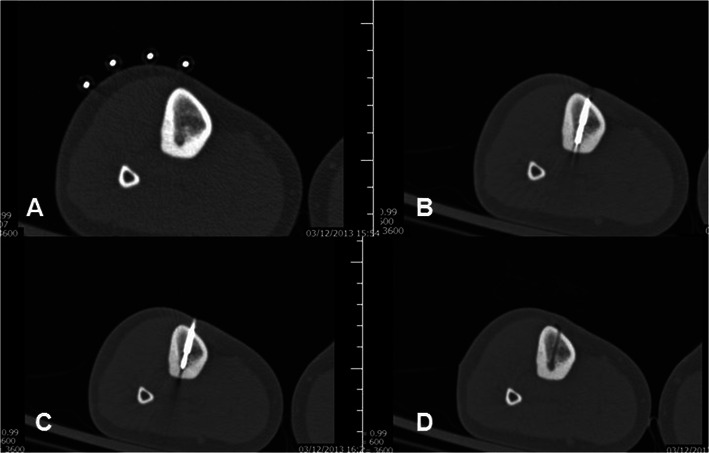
Osteoid osteoma in the tibia: a CT axial scan: metallic markers were placed on the patient’s skin. b CT axial scan: Once the trocar reaches the lesion, the needle is removed and a bone biopsy needle is inserted coaxially for sampling to verify the diagnosis. c CT axial scan: Then, the radiofrequency electrode is inserted into the lesion coaxially and an ablation protocol specific for osteoid osteoma is performed. d CT axial scan: Follow-up post trocar removal. Notice the trocar tract, which ends up in the lesion
